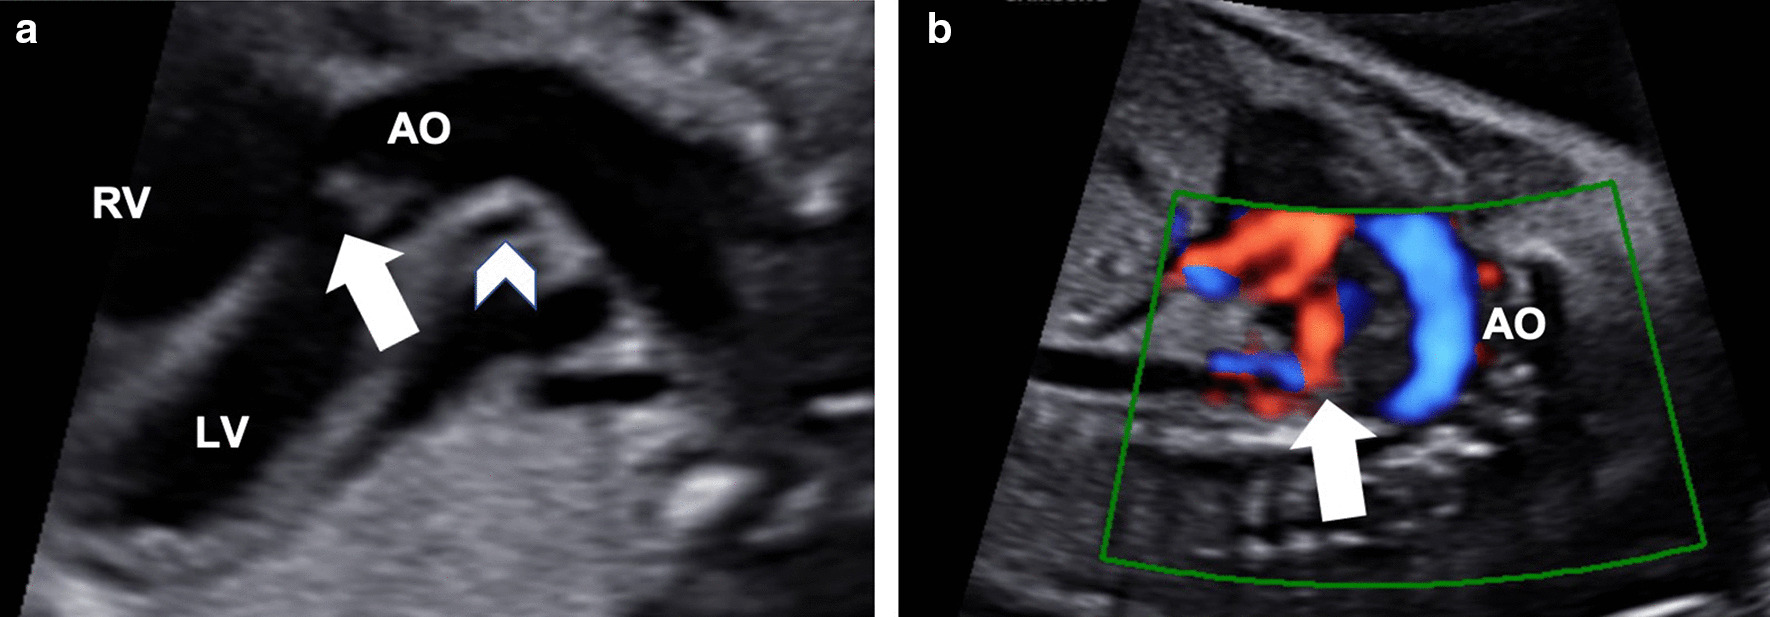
Prenatal echocardiogram showing a large ventricular septal defect, an overriding aorta (arrow), and an atretic main pulmonary artery (arrowhead) (a) and multiple aortopulmonary collateral arteries (b)
